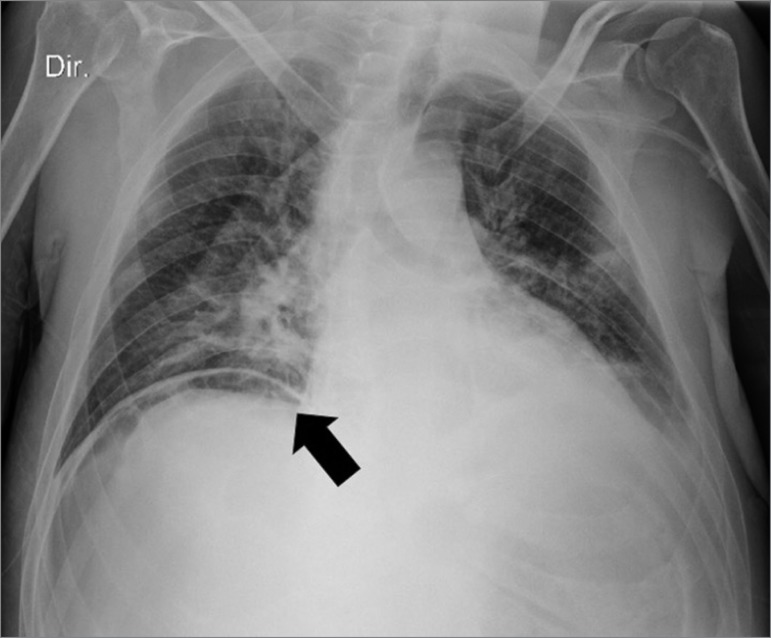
Patient’s thoracic radiography showing a pneumoperitoneum (black
